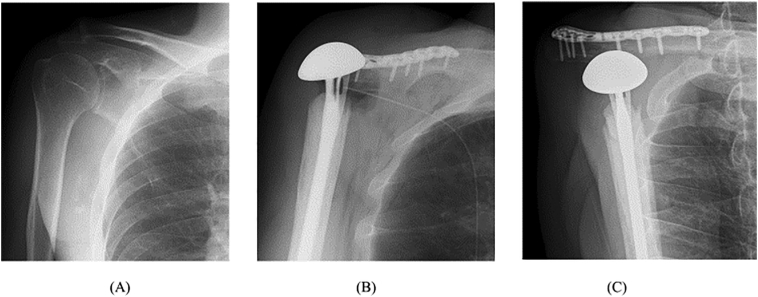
Preoperative and postoperative radiographs of a 56-year-old man's right shoulder. (A) Preoperative frontal view with suspected osteolysis of the scapula. (B) Frontal view after shoulder girdle resection, with shoulder stability provided by the clavicle-locking plate, Nesplon tape, and a proximal humeral prosthesis. (C) Postoperative frontal view implant head slightly more drooped than immediately after surgery, but stable.
